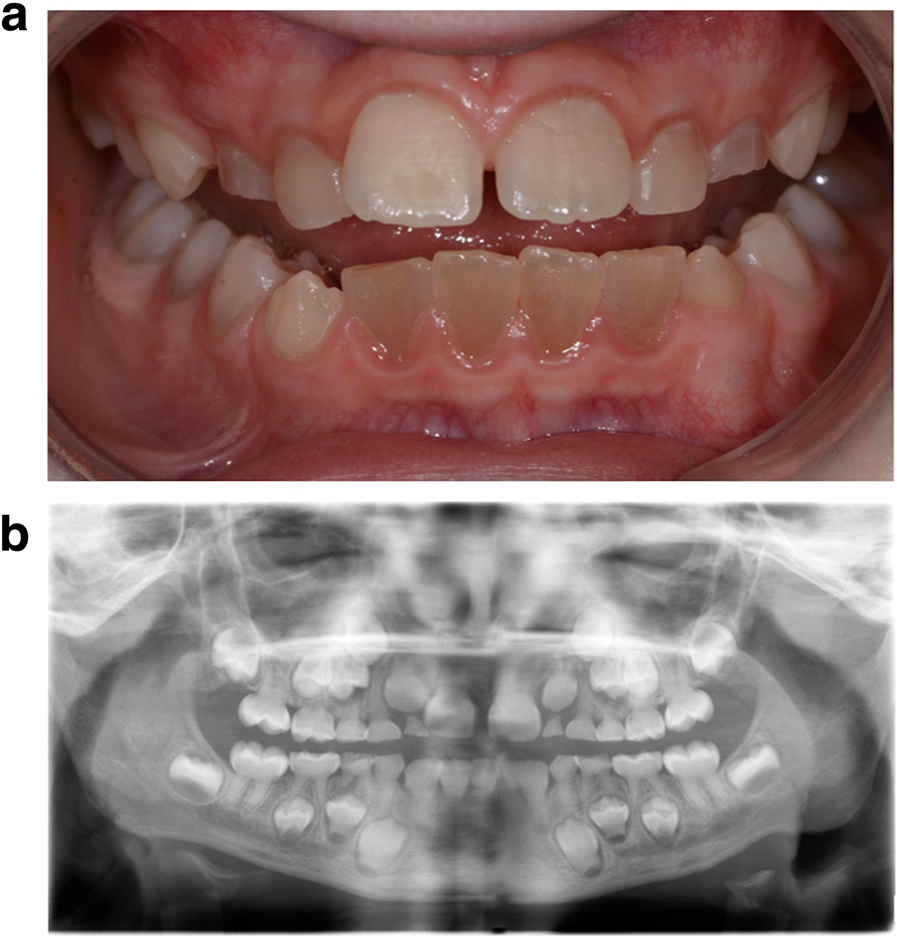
a A 10-year-old boy presenting with dentinogenesis imperfecta (DGI) type II in the mixed dentition. In the permanent dentition, the mandibular incisors are most severely affected. b Radiographic findings of DGI in the same boy at 6 years of age. Marked cervical constriction, varying degree of pulpal obliteration, and short roots are visible
